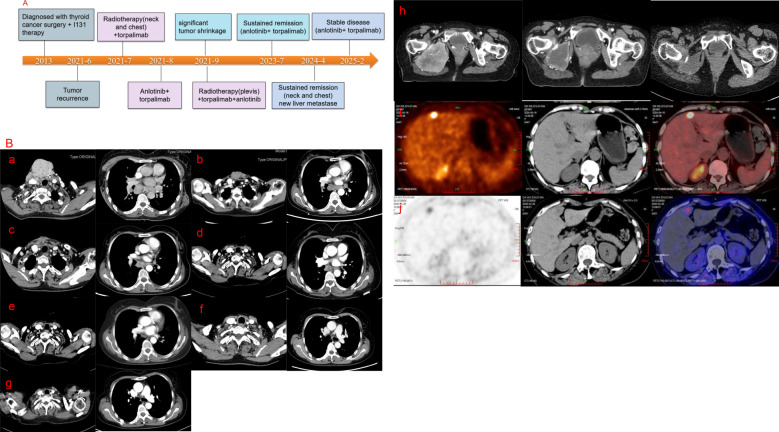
(A) Timeline of treatment. (B) A series of CT images of the patient. a-g, CT images of the patient’s neck and chest. (a) June 2021 (b) September 2021 (c) November 2021 (d) April 2022 (e) July 2023 (f) January 2024 (g) February 2025. (h) CT images of the bone metastasis, and the examination time from left to right is September 2021, November 2021, and April 2022, respectively. Figure (i) shows the PET-CT image of the patient’s liver metastase in April 2024.Figure (j) shows the PET-CT image of the patient’s liver metastase in February 2025.
